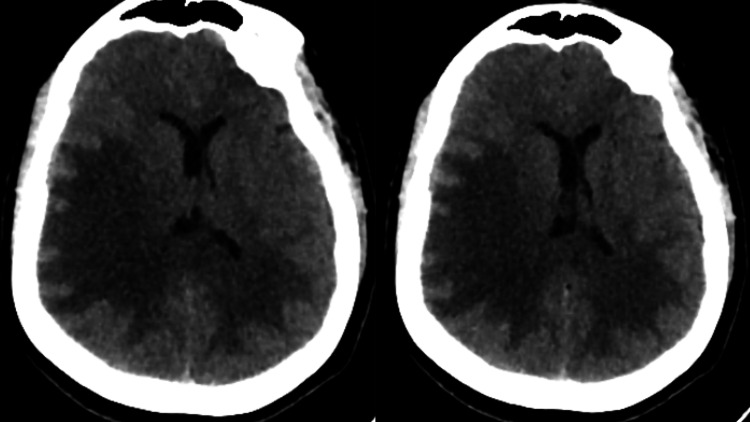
CT of the brain showing new white matter hypodensity involving the right cerebral hemisphere, causing a mass effect and midline shift. The left-sided hypodensity also appears to have increased June 10, 2024 CT: computed tomography
